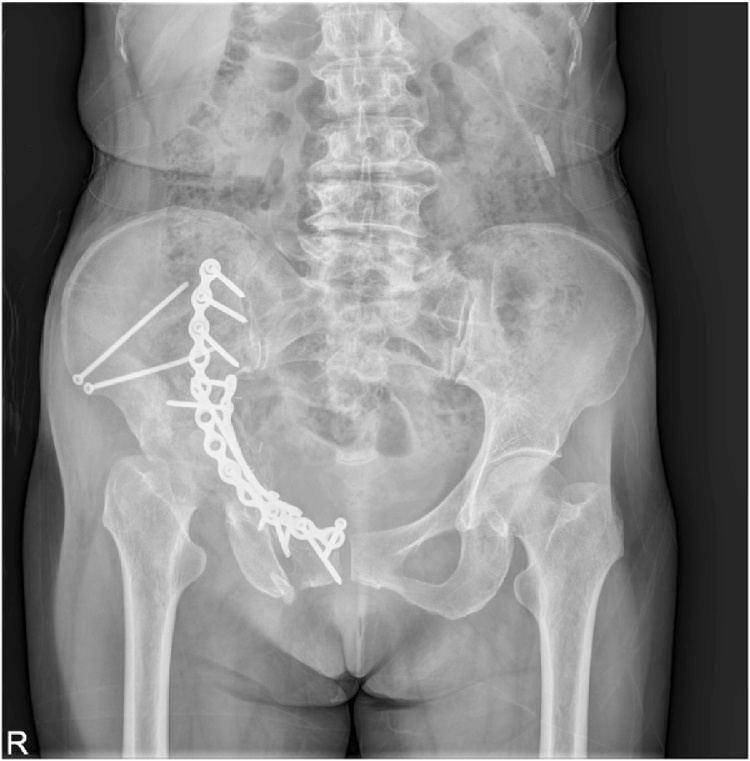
1 month post–operative radiograph of the pelvis showing normal head of the femur.
